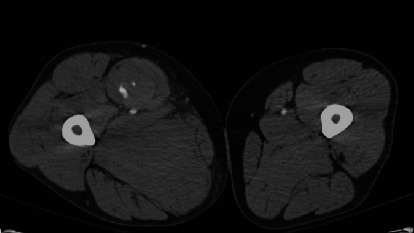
An axial CTA image of bilateral thighs demonstrating a hematoma in the sartorius of the right thigh.
